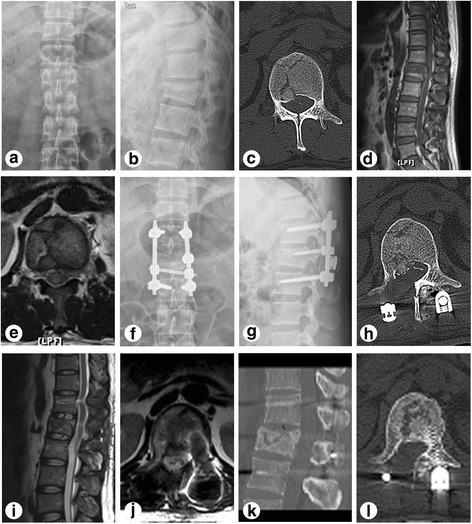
Preoperative, postoperative, and follow-up radiographs of the patient who had thoracolumbar burst fractures with greenstick lamina fractures managed by the open book laminectomy approach (case 3). a–e X-ray, CT, and MRI before the posterior pedicle screw fixation. f–h X-ray and CT at 3 days after operation. i, j MRI at 3 months after operation. k, l CT at 6 months follow-up
